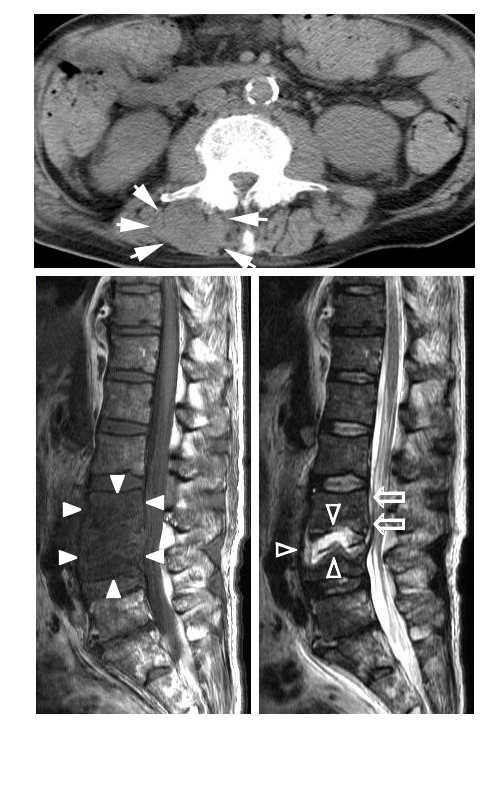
Lumbar CT scan and MRI: CT demonstrates swelling ( *white allow:* ↑) of the left paraspinal muscle around the L2 level. (A) MRI reveals spondylitis (*white allow head:* ▴) lesions involving the L3-L4 vertebrae (B) with ventral contiguous epidural (*framed allow: ⇑*) and disc (*framed allow head: △*) involvement (C).
